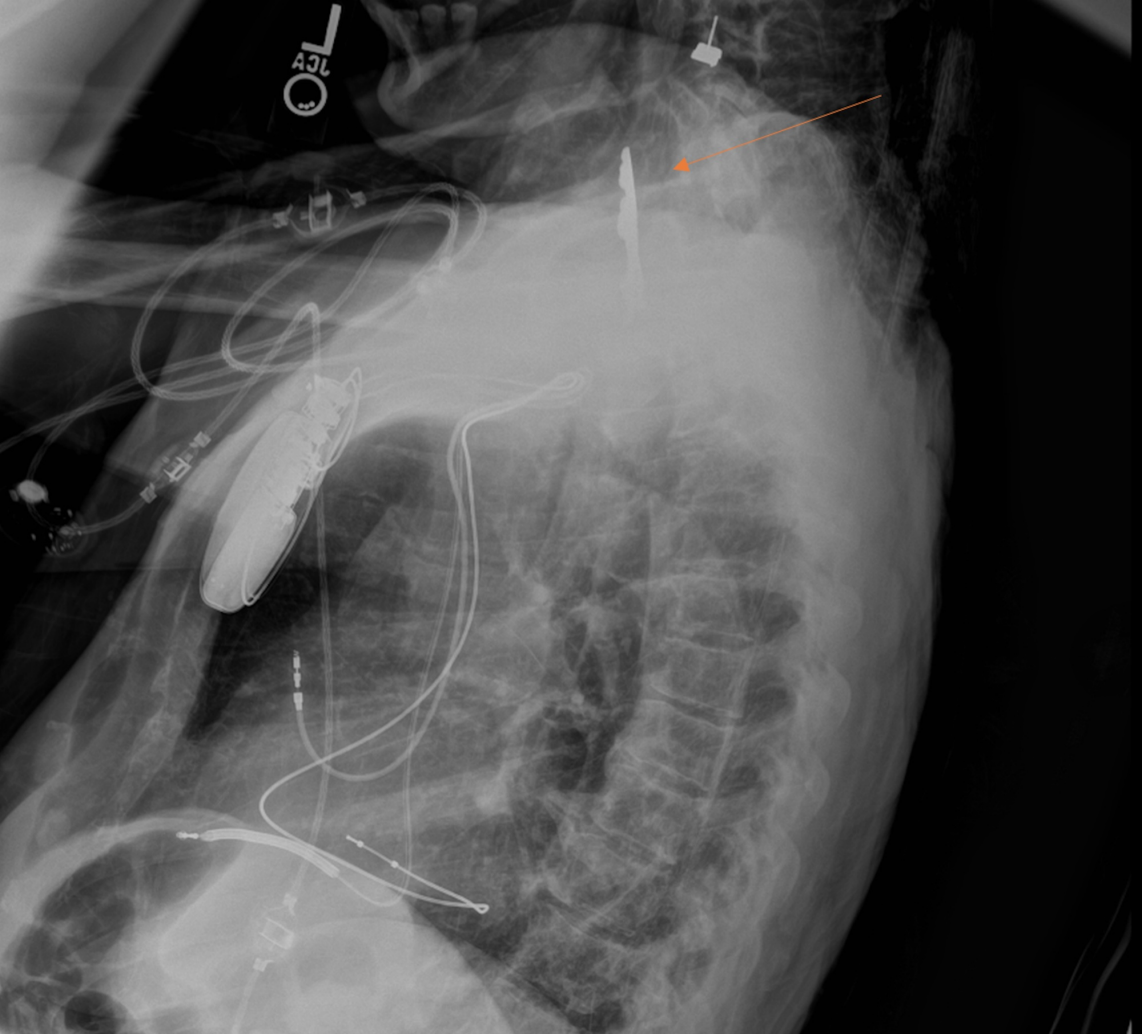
Lateral chest radiography demonstrating crucifix inferior to the epiglottis and posterior to the trachea consistent with a proximal esophageal foreign body (orange arrow).
